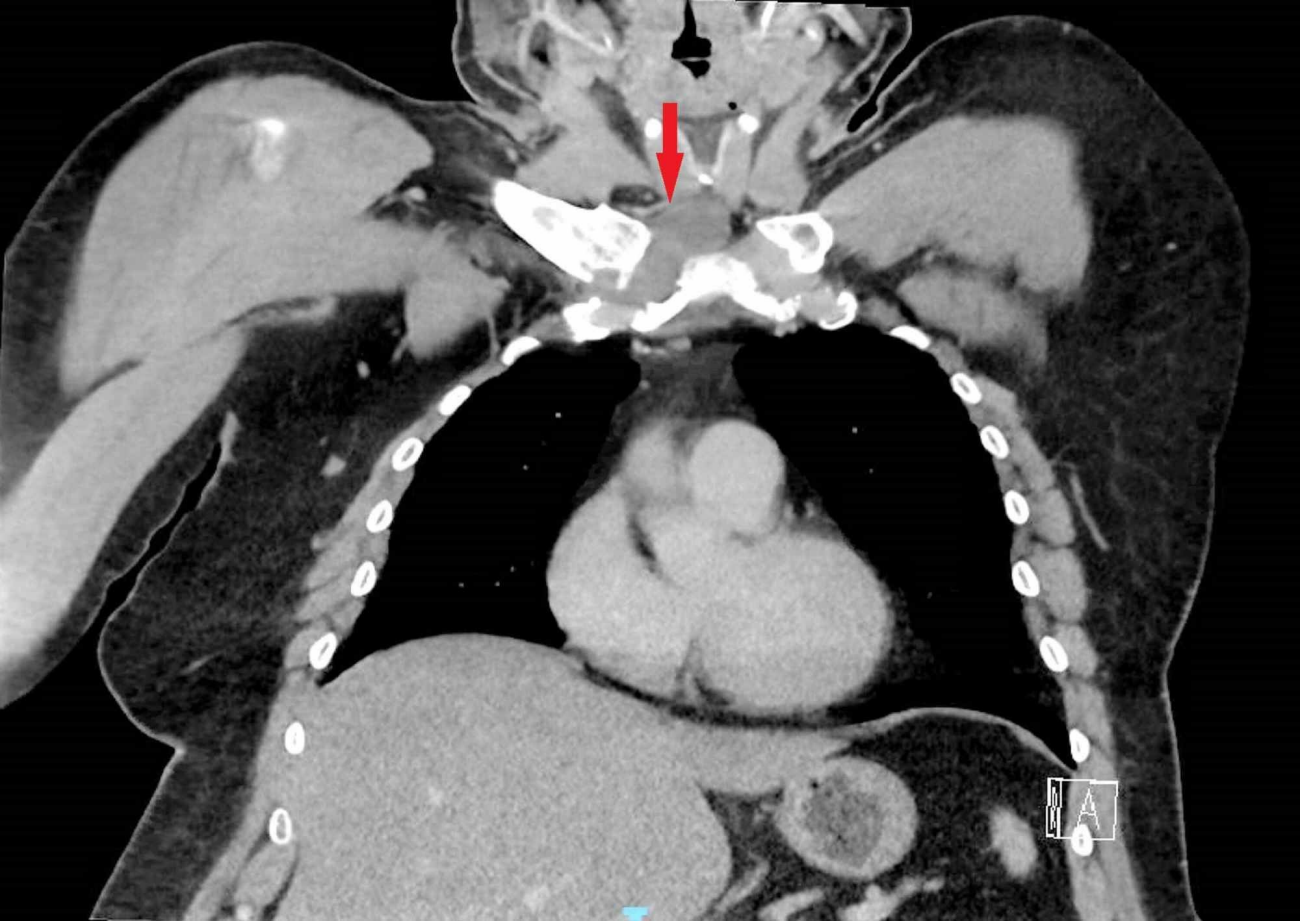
CT of the chest showing right sternoclavicular septic joint with osteomyelitis at the head of the right clavicle's connection with the manubrium (red arrow) CT: computed tomography
